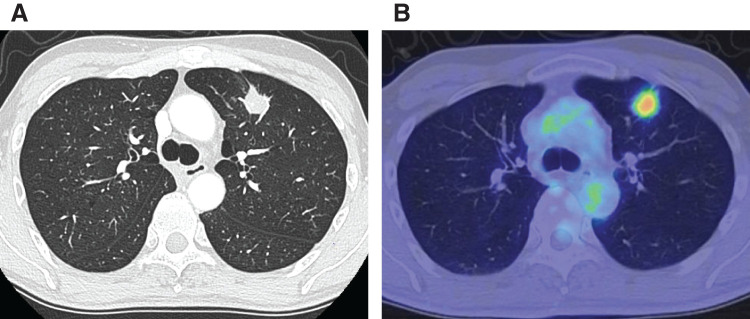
(A) Chest CT showing a 23 × 20-mm nodule in the upper lobe of the left lung. (B) PET-CT showing increased fluorodeoxyglucose uptake in the same lesion (maximum standardized uptake value, 4.89).
